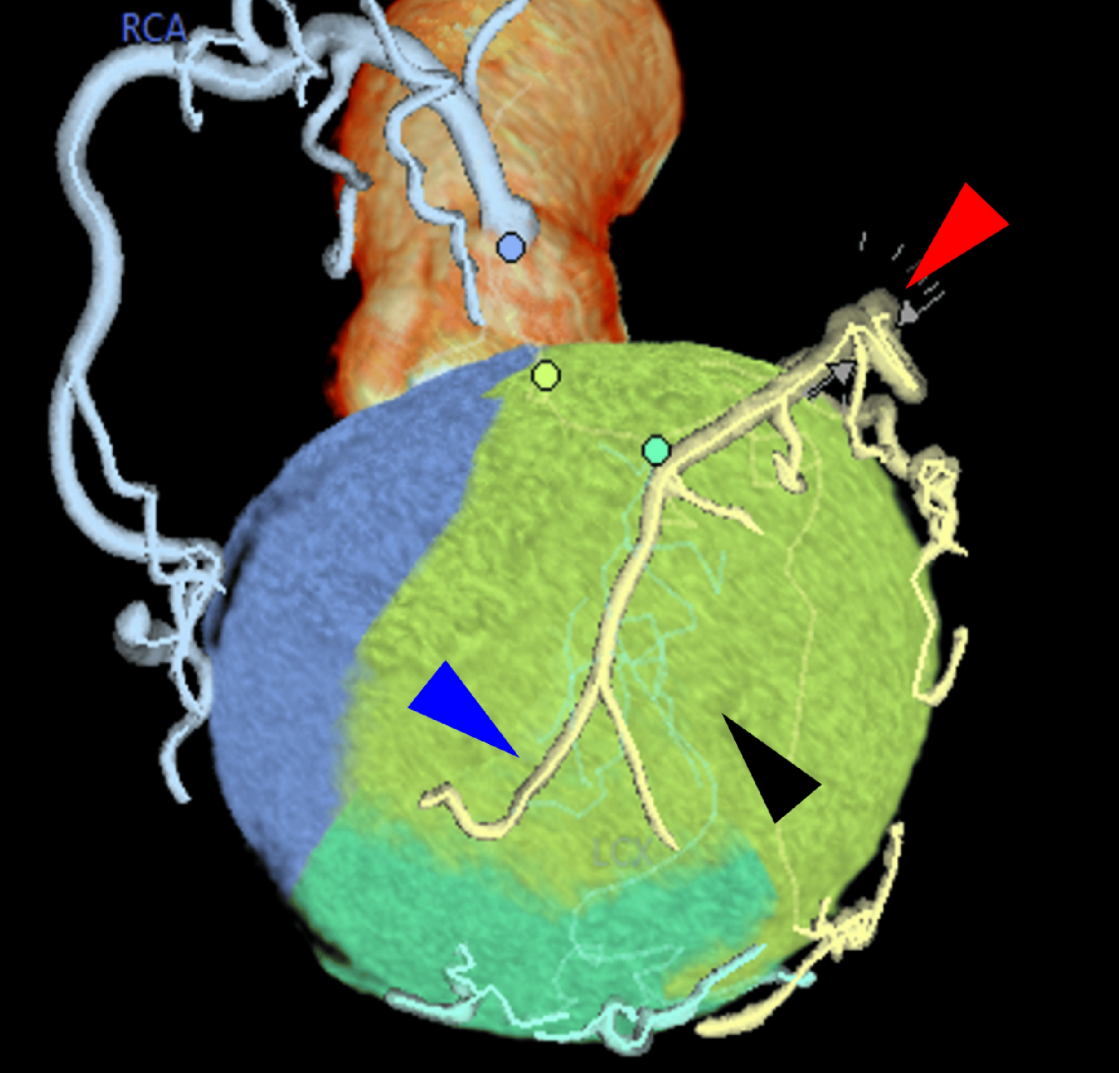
Left anterior oblique caudal to cranial view of the CTA scan with the perfusion Red arrowhead: LAD artery; Black arrowhead: Apex of the heart; Blue arrowhead: PDA ascending in the posterior interventricular groove CTA: CT angiography; LAD: Left anterior descending; PDA: Posterior descending artery
